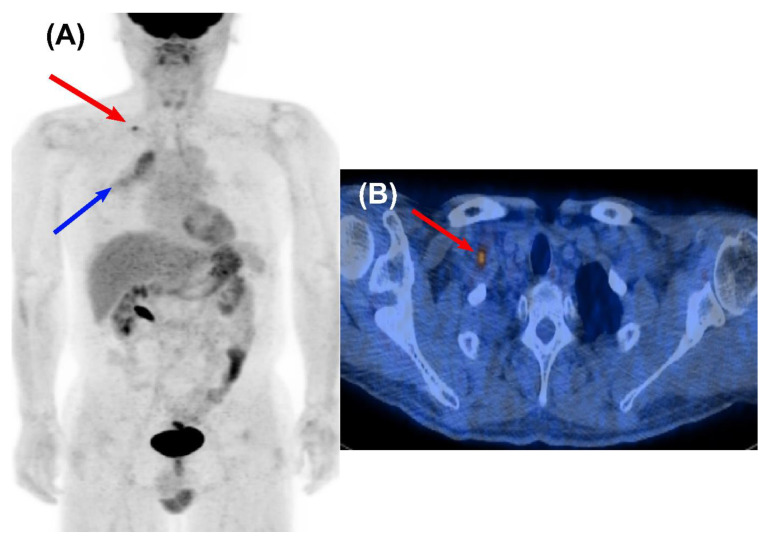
Loco-regional recurrence detected by surveillance FDG PET/CT in a 54-year-old male who underwent curative surgery for NSCLC. Focal FDG uptake was noted in the right supraclavicular lymph node on a maximum intensity projection (MIP) (A) and a transaxial fused PET/CT image (B) obtained at 7 months after curative resection. The lesion was confirmed as biopsy-proven recurrence (red arrow). He survived for 60 months after localized treatment for the lesion. Diffusely increased FDG uptake on the right upper lung field indicates radiation pneumonitis (blue arrow).
